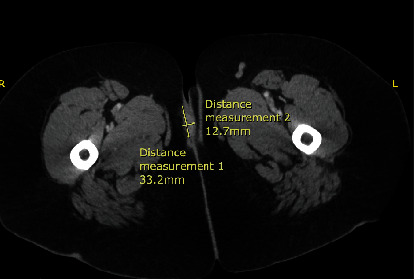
CT scan of the fungating mass with surrounding edema near the right vulvar region, near the groin crease.
